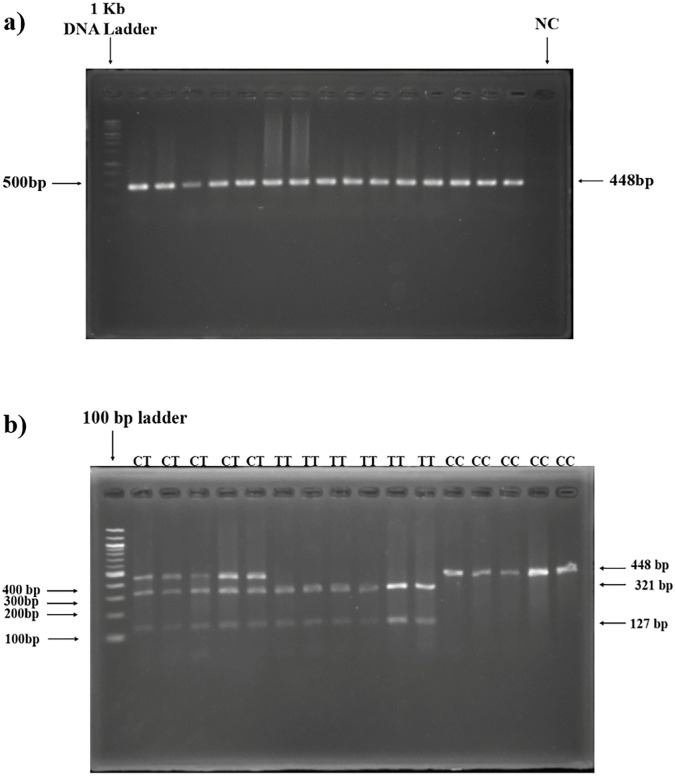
Visualization of PCR and restriction digested products (rs12794714). a) PCR product of the desired 448 bp *CYP2R1* gene segment amplicon targeting rs12794714, b) Restriction digestion products of CC (448 bp), CT (448 bp, 321 bp and 127 bp) and TT (321 bp and 127 bp) genotypes.
